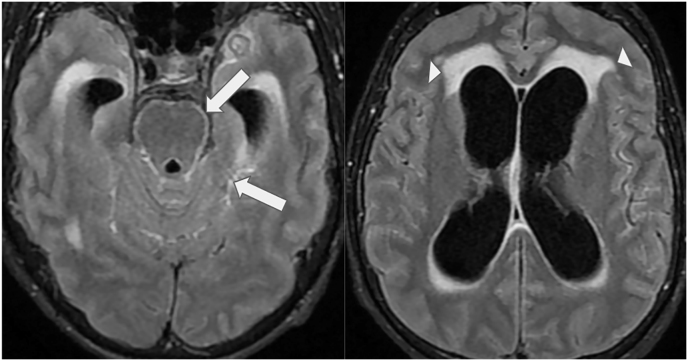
Brain MRI. Axial contrast-enhanced Fluid Attenuation Inversion Recovery (FLAIR) sequence shows linear areas of high signal intensity in the leptomeningeal surface of the brainstem and medial temporal cortex (arrows), enlarged supratentorial ventricles and adjacent transependymal white-matter edema (arrowheads).
